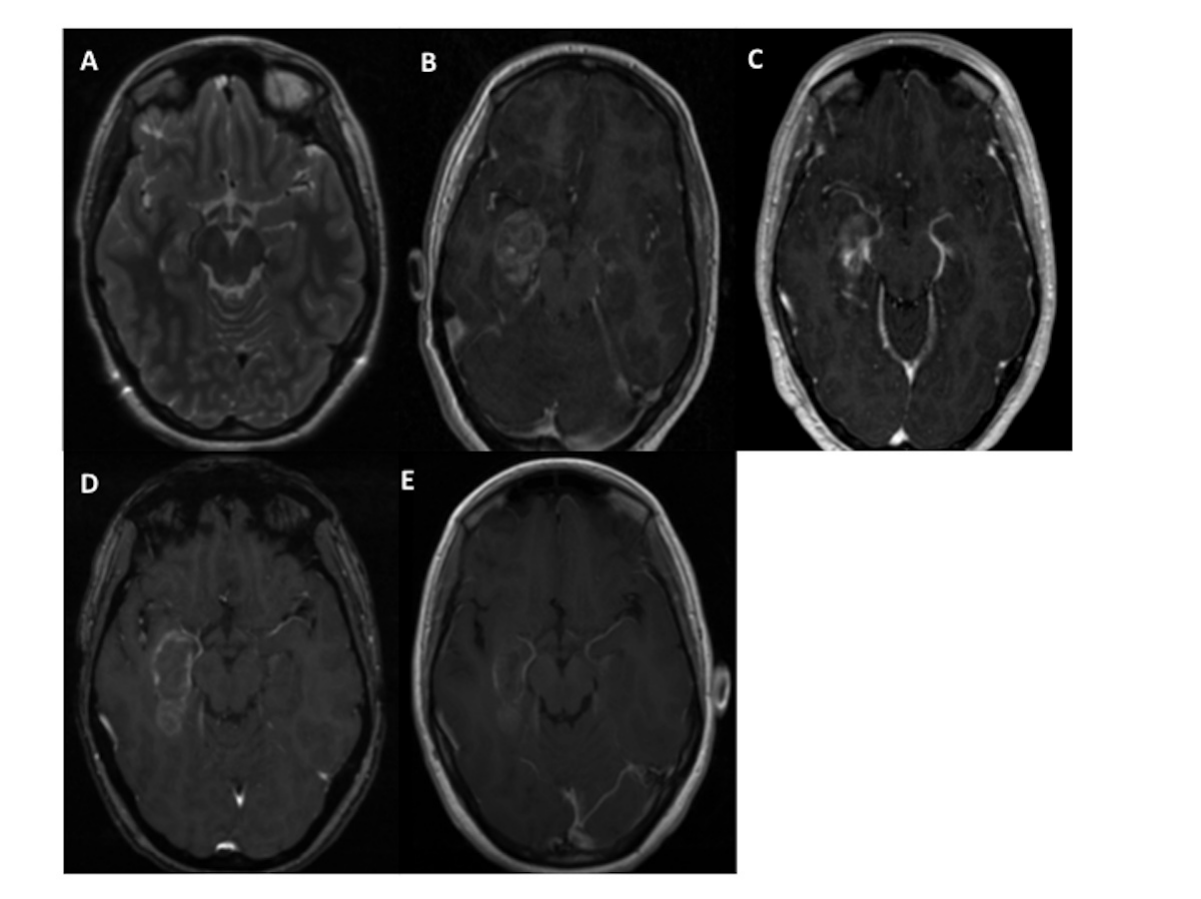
Radiographic Characteristic of the Ablation Cavity over Time A: Pre-operative T2 sequence MRI for patient G, showing right-sided mesial temporal sclerosis. B: IPA time point, with strong contrast enhancement. C, D: 24PA and FPA time point respectively, ring enhancement persists. E: >3MPA time point demonstrating decreased contrast enhancement and beginnings of cavitation at the center of ablation bed. Immediate post-ablation (IPA), 24 hours post-ablation (24PA), first follow-up post-ablation (FPA), and greater then 3 months follow-up post-ablation (>3MPA)
